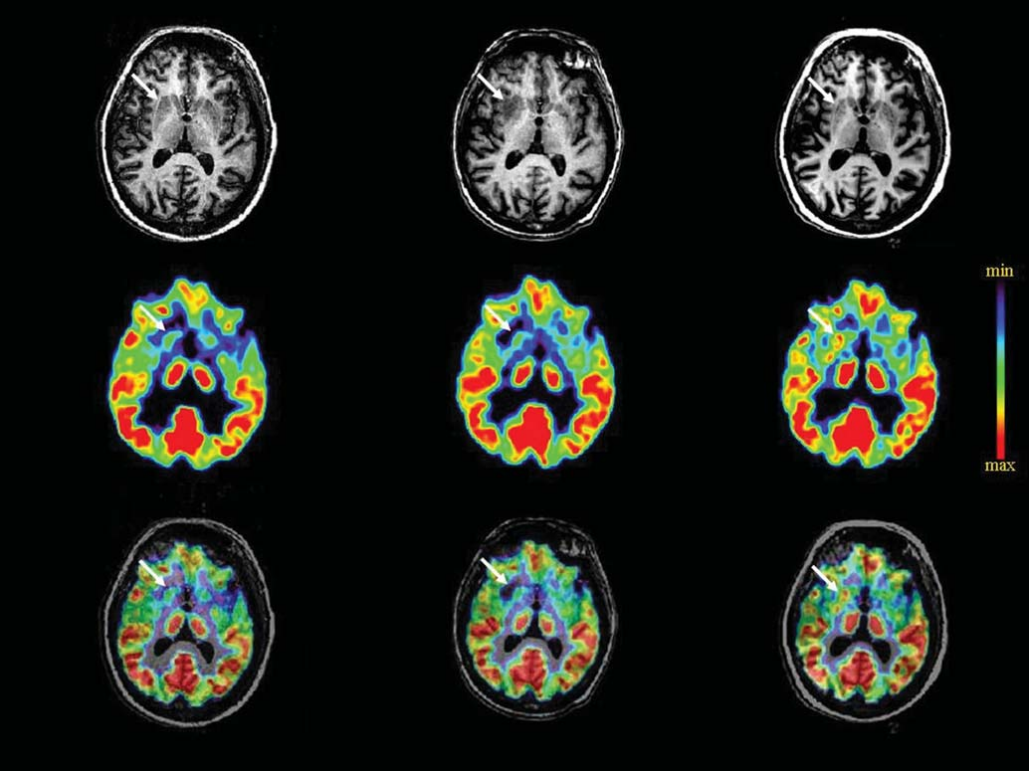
Brain imaging of the rejection process in patient 3 and its reversion under treatment. Magnetic resonance imaging and metabolic activity using ^18^F-deoxyglucose before surgery (T0), during the rejection process (T1) and after 6 months of reinstated immunosuppressive treatment (T2) are shown separately (upper and middle panel, respectively), then co-registered (lower panel). The white arrow indicates the right striatum. The false colour scale shows levels of metabolic activities from lowest (min) to highest (max).
